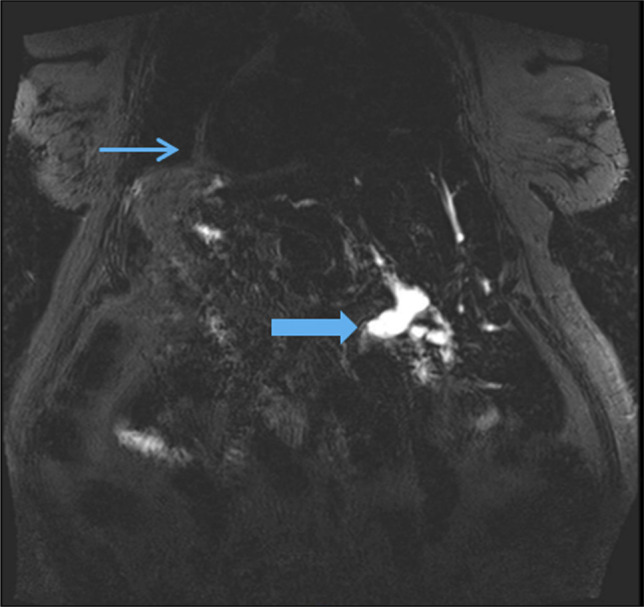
Coronal MRI demonstrates markedly dilated CBD with distal tapering and abrupt cutoff of the CBD with multiple filling defects (thick arrow). Situs inversus and dextrocardia (thin arrow) are seen. CBD, common bile duct; MRI, magnetic resonance imaging.
